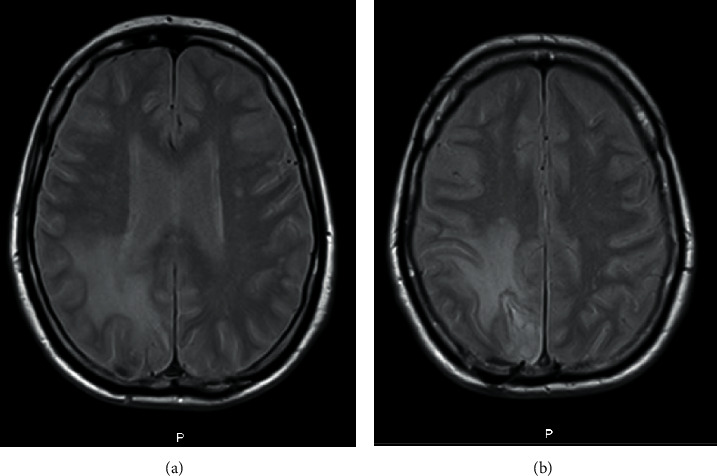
CE-MRI at 28 hospitalisation day.
